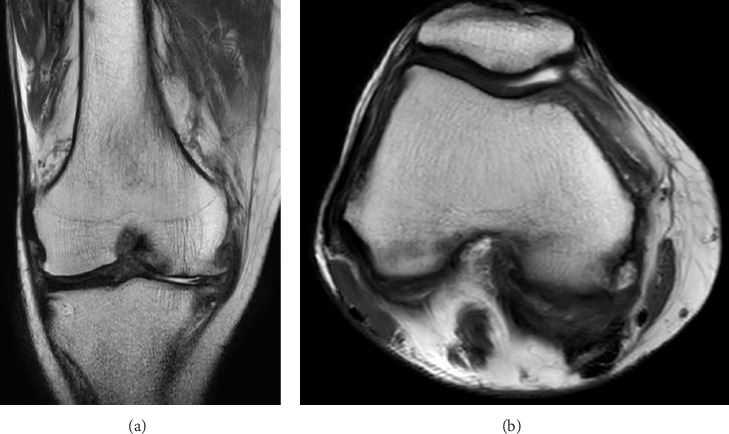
Coronal and axial magnetic resonance imaging of the right knee (T2WI high) after azacitidine treatment. Thickened synovium diminished after treatment (a, b).
